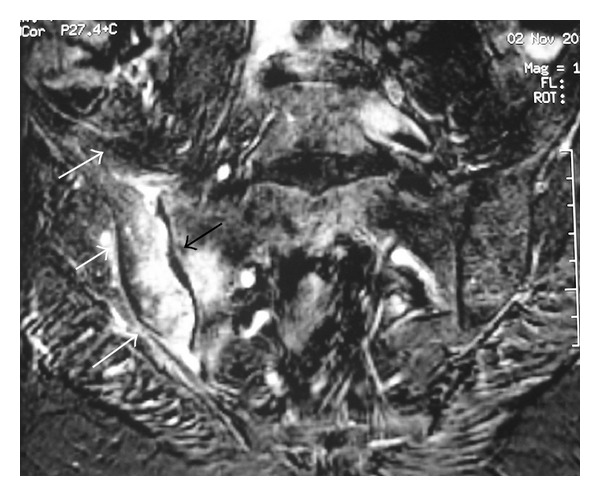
Post-contrast coronal T1-weighted MR image of the pelvis shows areas of diffuse enhancement of the right sacroiliac joint and the adjacent iliacus and gluteal muscles.
